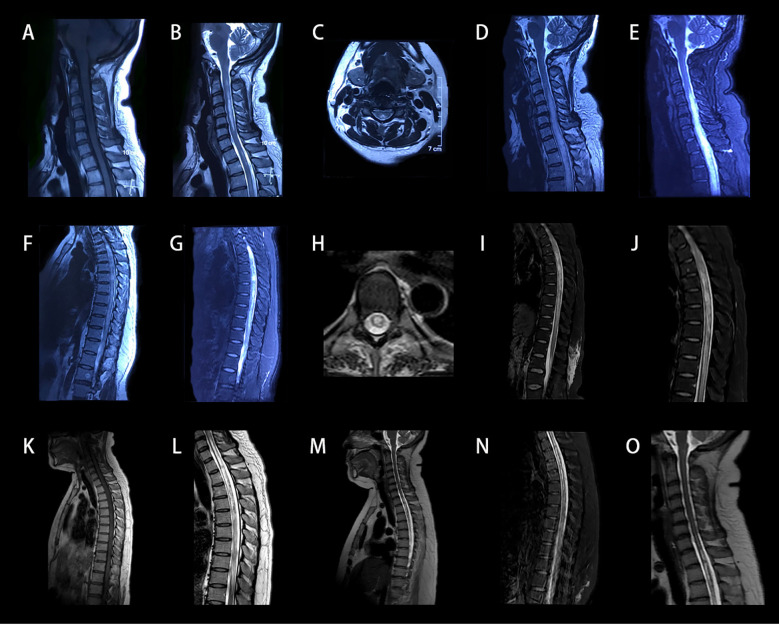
Cervical MRI performed during acute phase of disease. (A–C) Sagittal and axial T1 or T2-weighted images of cervical MRI scans at first-onset show posterior cord lesions along the C2-C6 levels and upper thoracic segments, without enhancement. (D–J) Cervical MRI shows longitudinally extensive abnormal spinal cord signal intensity with long T1-weighted (D, F) and long T2-weighted (E, G) at C2-T11 levels with swelling. The Gd-enhanced MRI showed significant enhancement of cervical and thoracic spinal cord lesions (H–J). (K, L) Following combined IVMP and IVIG therapy, MRI demonstrates reduction of spinal cord swelling with decreased hyperintense signal intensity extending from C6 to T10 levels. The Gd-enhanced MRI showed no significant enhancement of spinal lesions, but syringomyelia were present at C7-T2. (M–O) Follow-up MRI following first-line therapy demonstrates multi-focal patchy hyperintensities spanning T1-T10 on T2, and mild enlargement of syringomyelia compared to prior imaging, extending from C6 to T4. MRI, magnetic resonance imaging; Gd-enhanced, gadolinium enhanced; IVMP, intravenous methylprednisolone; IVIG, intravenous immunoglobulin.
